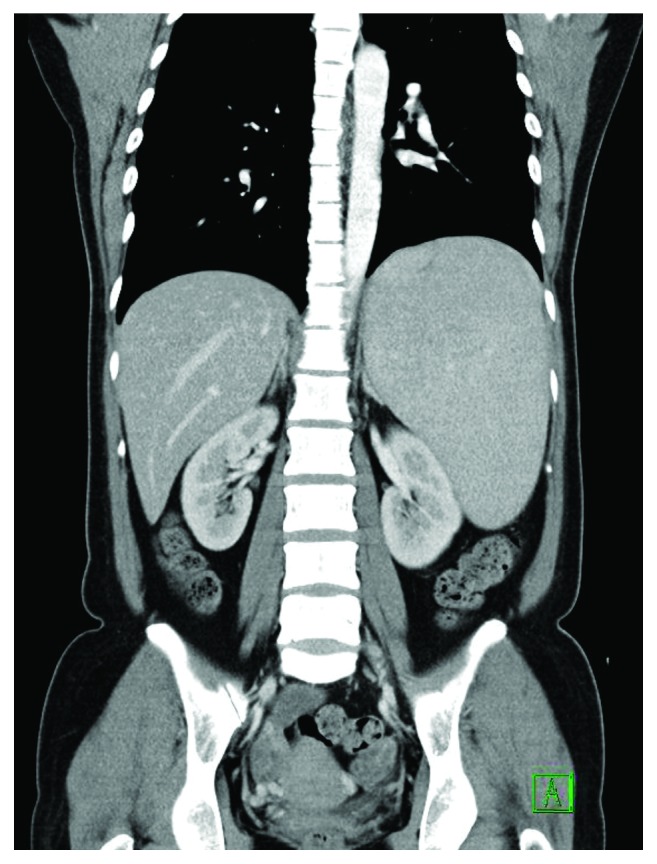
Computed tomographic coronal section showing hepatosplenomegaly.
